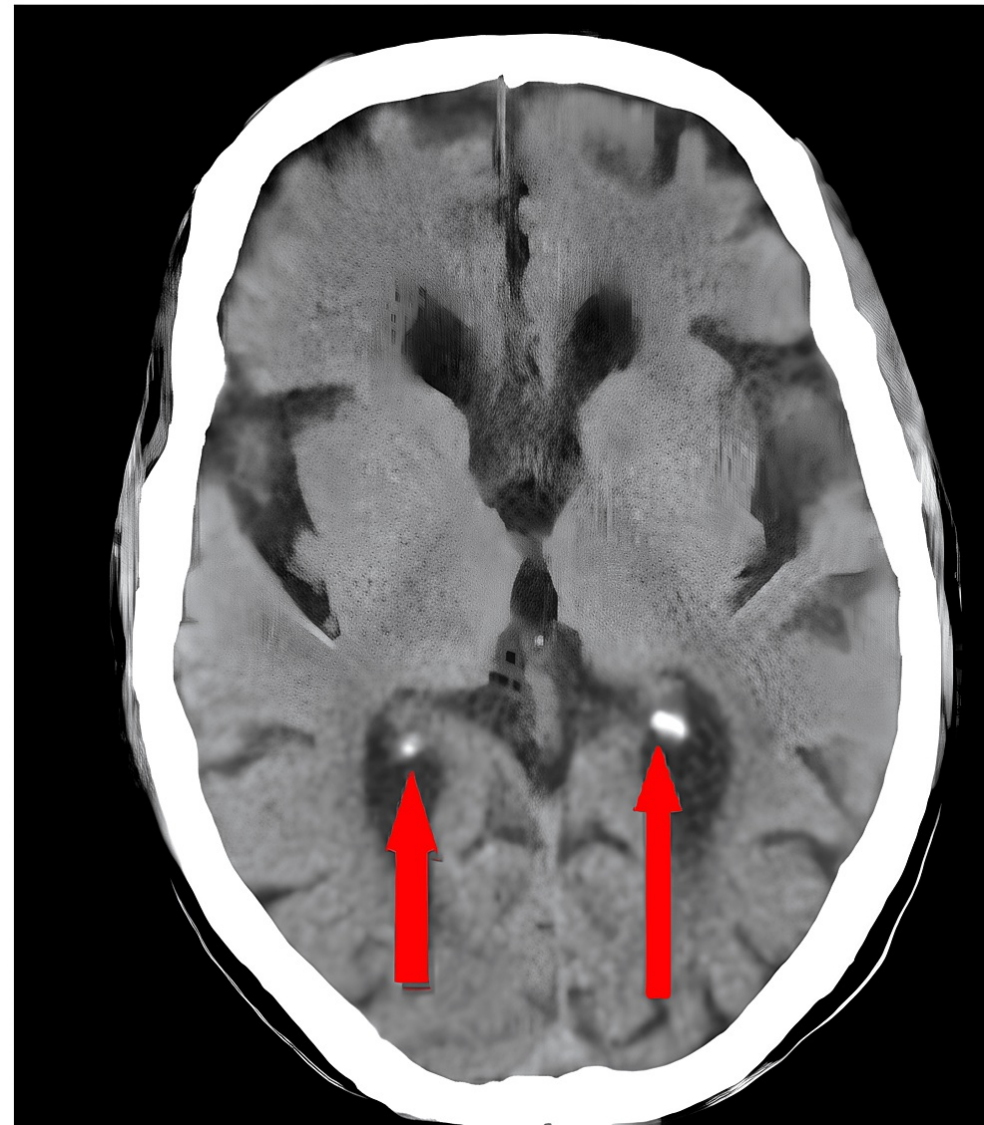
MRI findings of hyper-acute infarct in the posterior limb of the left internal capsule (red arrows)
